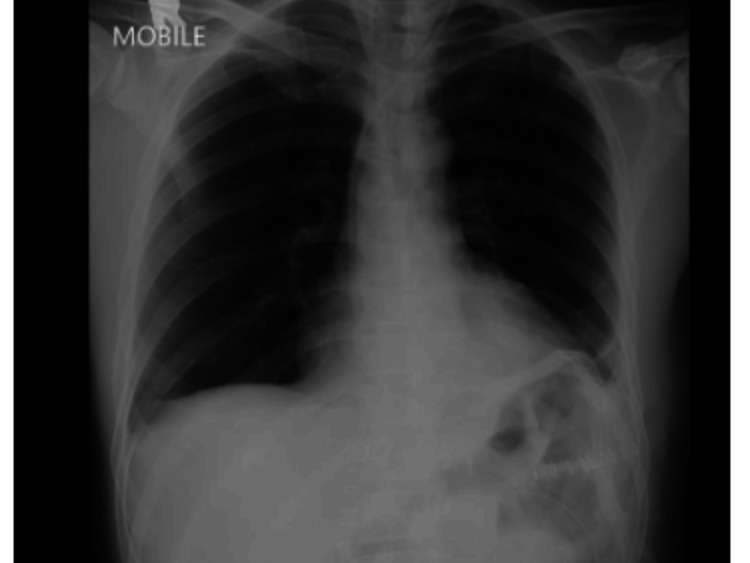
Chest radiograph on post-op day five, prior removing the chest drain and discharge
